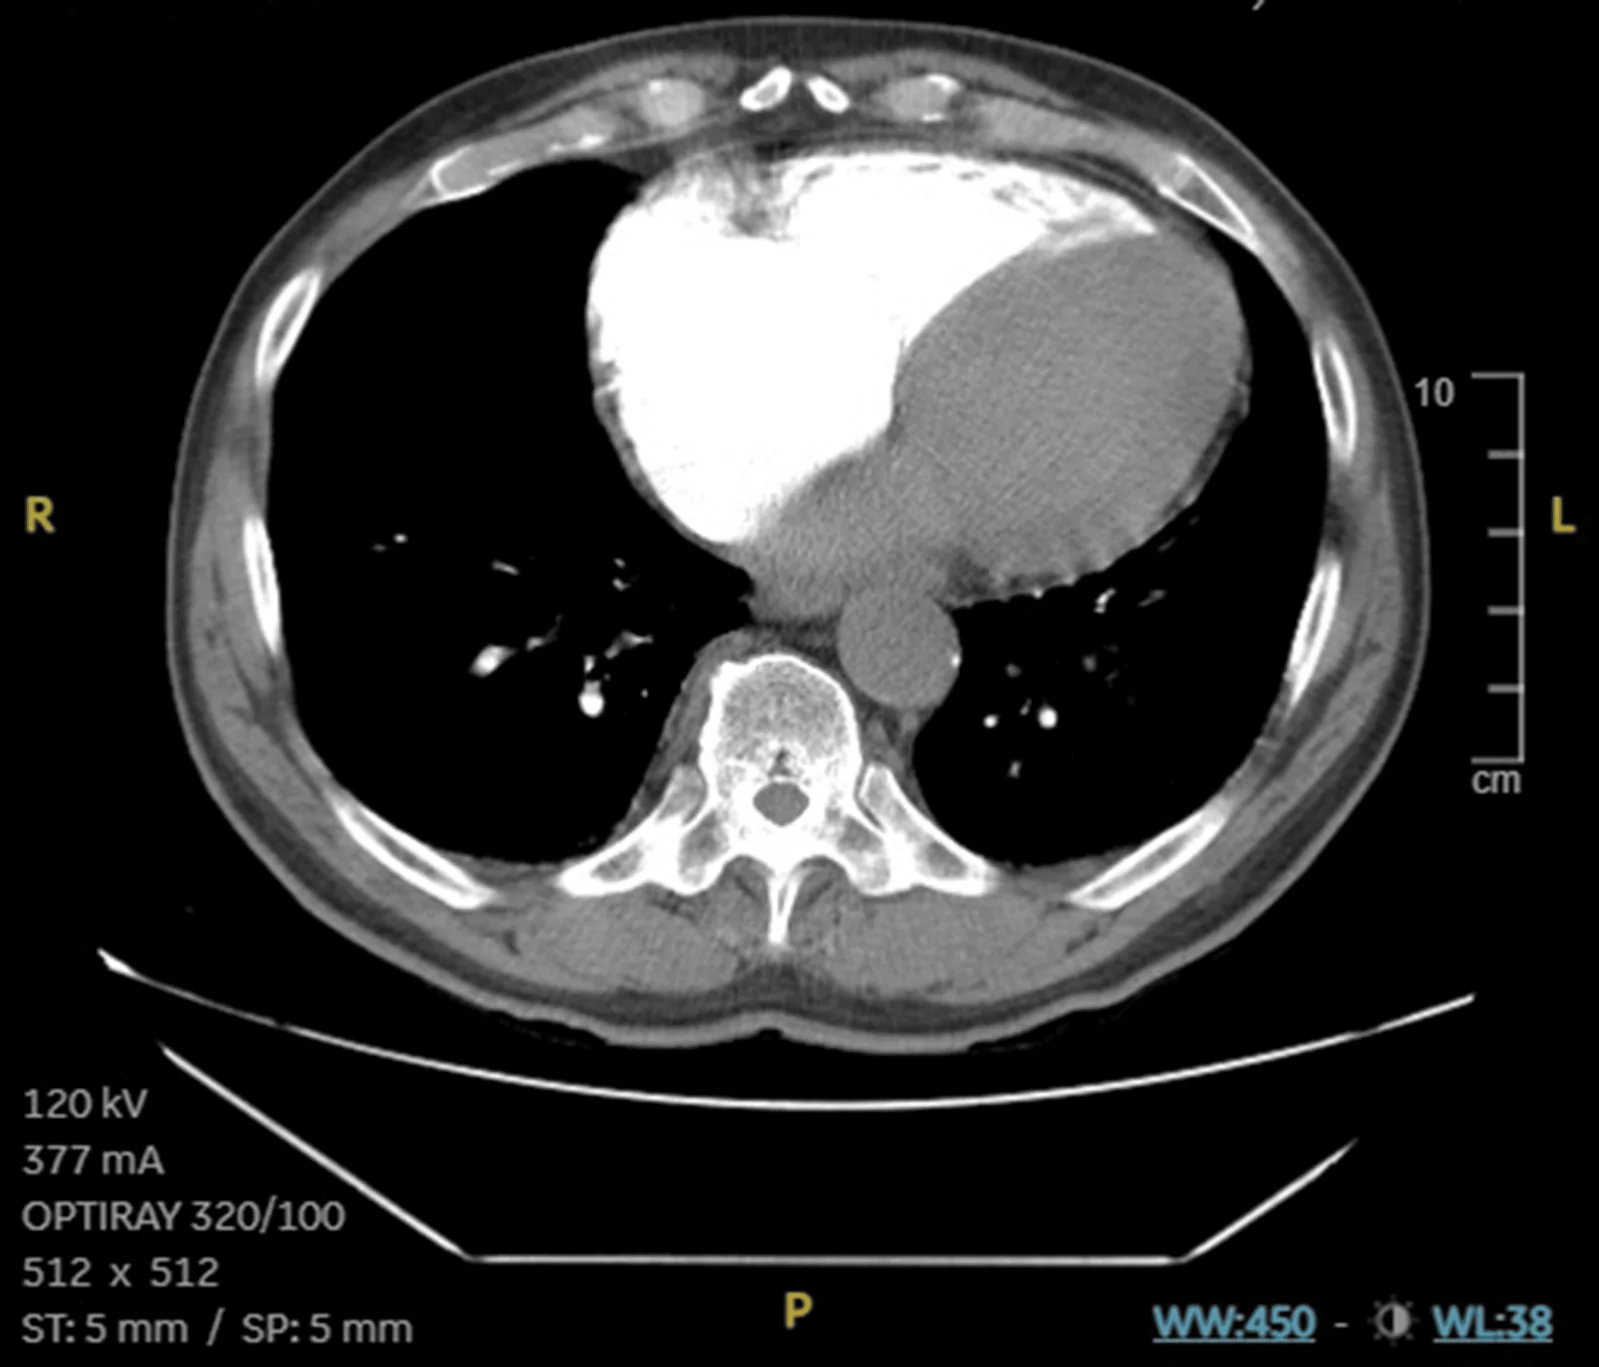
Prior contrast-enhanced CT chest demonstrating absence of the left lower lobe pulmonary mass Axial contrast-enhanced CT of the chest obtained eight months before presentation demonstrates emphysematous changes without evidence of a left lower lobe pulmonary mass. This prior study provides comparison with the subsequent development of the newly identified left lower lobe lesion CT: computed tomography
